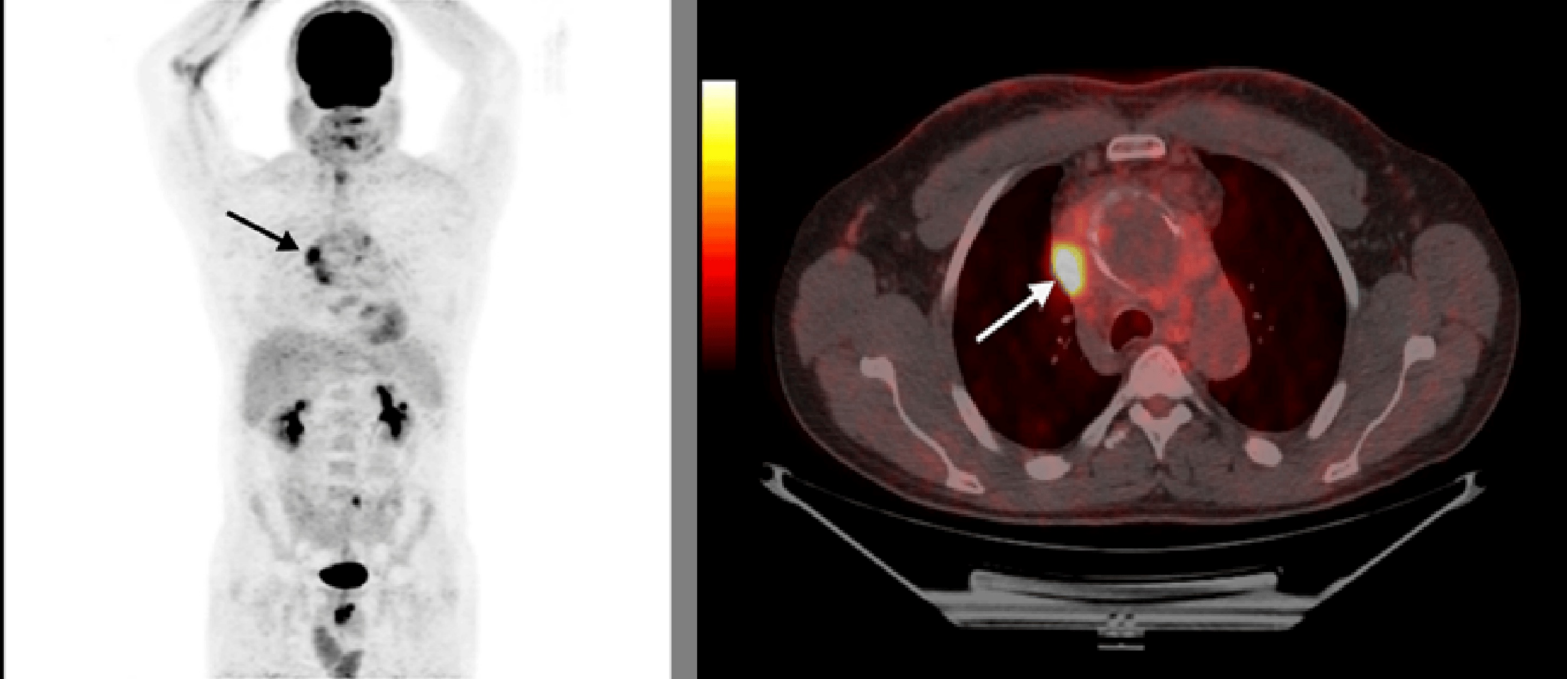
MIP (left) and PET/CT (right) images demonstrate marked right mediastinal hypermetabolism (arrows) related to SVC thrombosis and inflammation MIP: Maximal inspiratory pressure, PET/CT: Positron emission tomography-computed tomography, SVC: Superior vena cava
